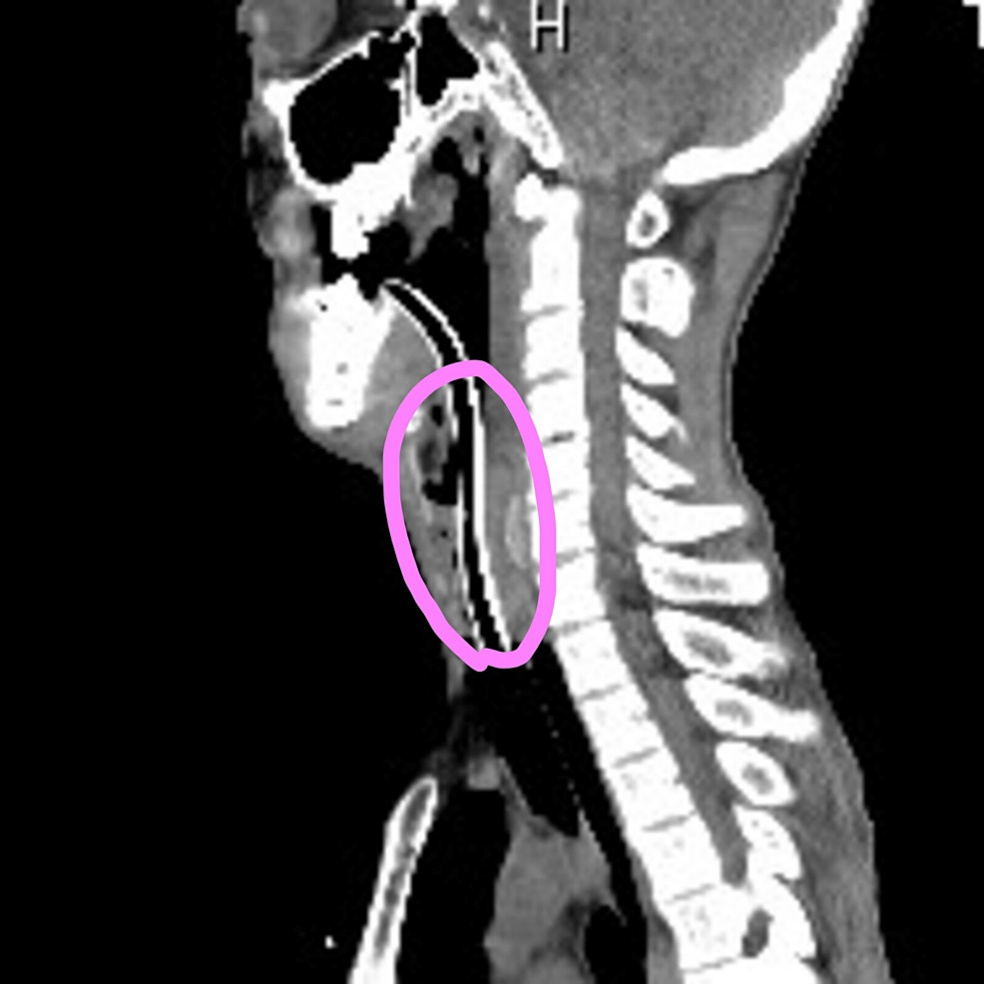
Cervical CT, lateral view. Post-return of spontaneous circulation CT reveals marked stenosis (50 mm in length) of the trachea below the glottis.
